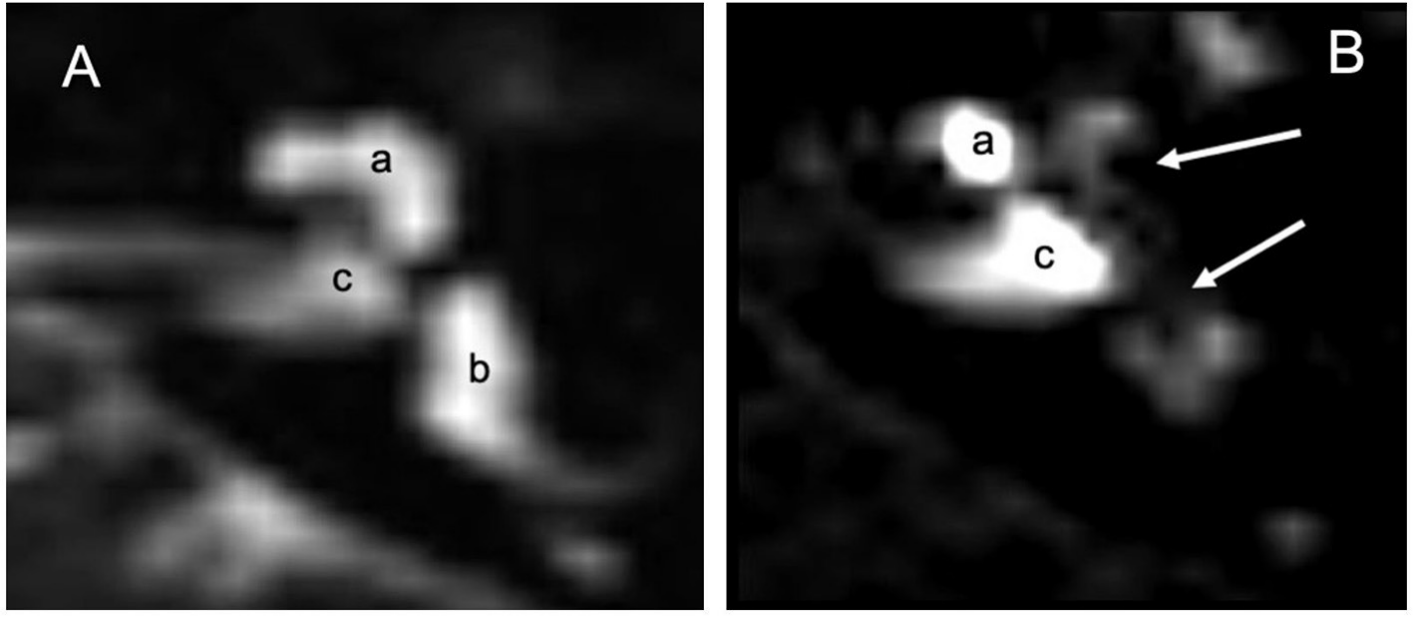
MRI 3 Tesla: (A) MRI in T2 without contrast means: labyrinthine fluids including peri and endolymphatic spaces are observed (a) cochlea, (b) vestibular spaces and CSL and internal auditory canal (c). (B) 24 h after infiltration with the Flair technique, the contrast shows absence of gadolinium in the apical gyrus of the cochlea and vestibule (white arrows). Gd illuminates part of the cochlea (a) and the internal auditory canal (c) which seems to be the main way of diffusion of gadolinium toward the liquor spaces.
